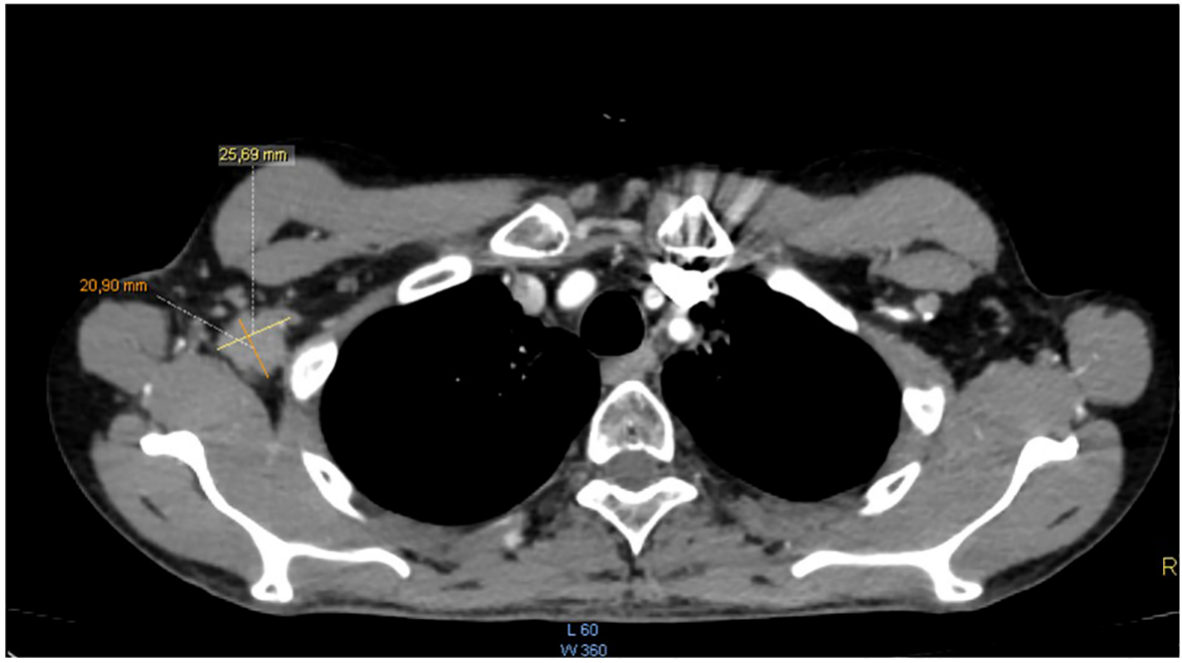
Axial CT scan with contrast injection: right axillary adenopathy measuring 25*20mm.
